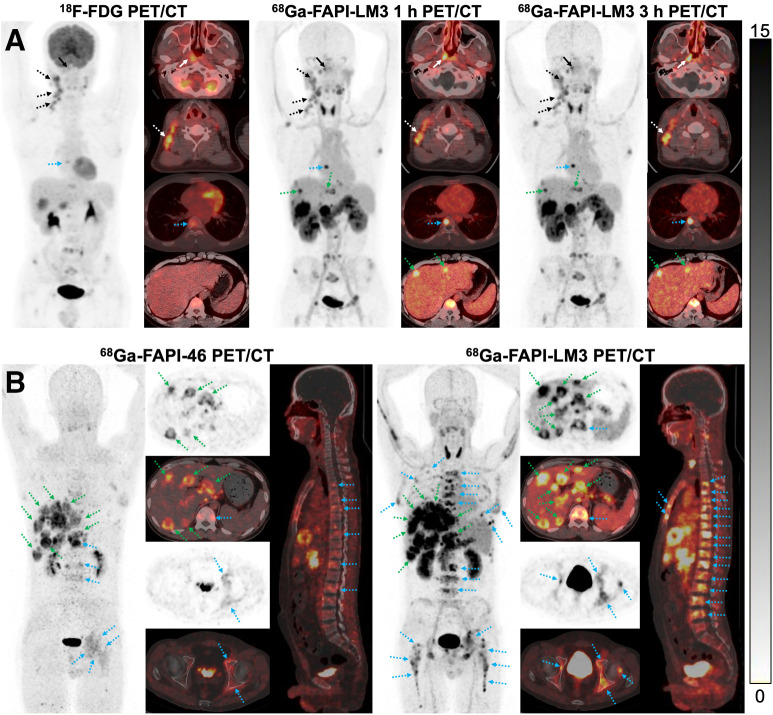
(A) PET/CT imaging findings in 32-y-old treatment-naïve patient with NPC. Both ^18^F-FDG and ^68^Ga-FAPI-LM3 PET/CT showed abnormal activity in primary tumor (solid white arrows), regional lymph node (dotted white arrows), and bone (dotted blue arrows). Additional liver metastases with intense activity (dotted green arrows) were observed on ^68^Ga-FAPI-LM3 PET/CT. However, these lesions were not visualized on ^18^F-FDG PET/CT. (B) PET/CT imaging findings in 44-y-old patient with metastatic NPC. ^68^Ga-FAPI-LM3 PET/CT revealed more lesions than did ^68^Ga-FAPI-46 for lymph node, liver (dotted green arrows), and bone (dotted blue arrows) metastases, with higher uptake.
